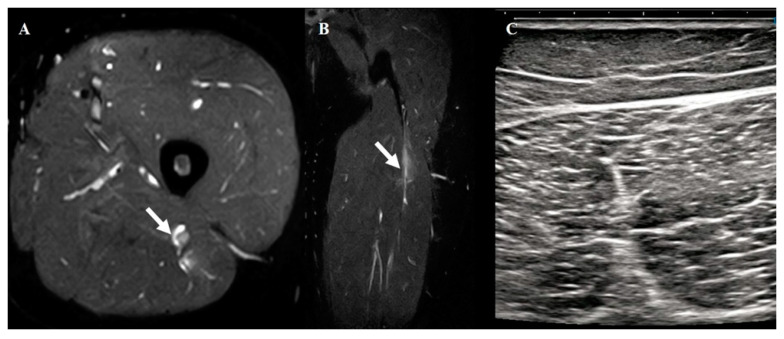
MRI findings of biceps femoris musculotendinous junction injury. Axial (A) and coronal (B) views of grade 1. White arrows indicate injured areas. (C) Short-axis ultrasonography findings in same case did not reveal any injury.
